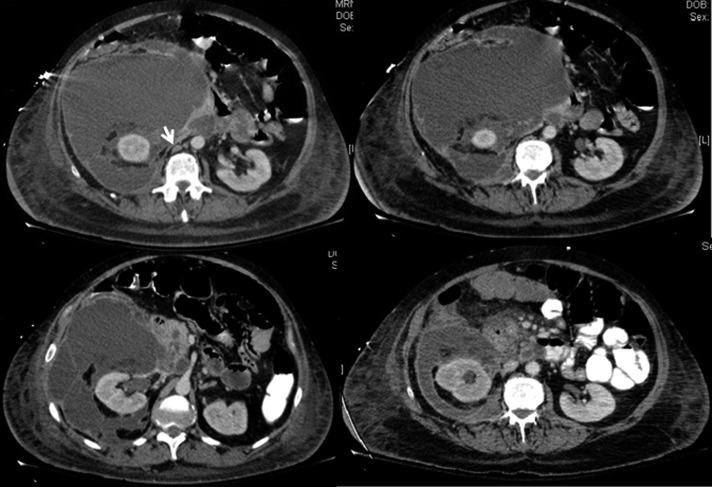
Abdominal computed tomography showing large amounts of retroperitoneal fluid involving the right perinephric spaces with kidney displacement. The arrow points to compression of the inferior vena cava secondary to mass effect from walled-off pancreatic necrosis.
